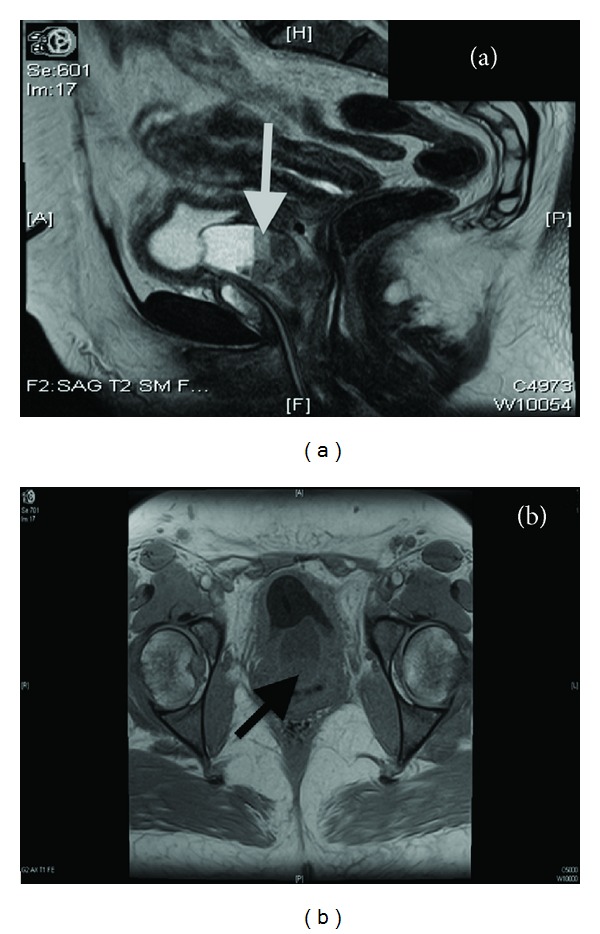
Magnetic resonance imaging shows 4.4 cm × 3.2 cm × 3.6 cm nodular enhancing mass concerning malignancy.
